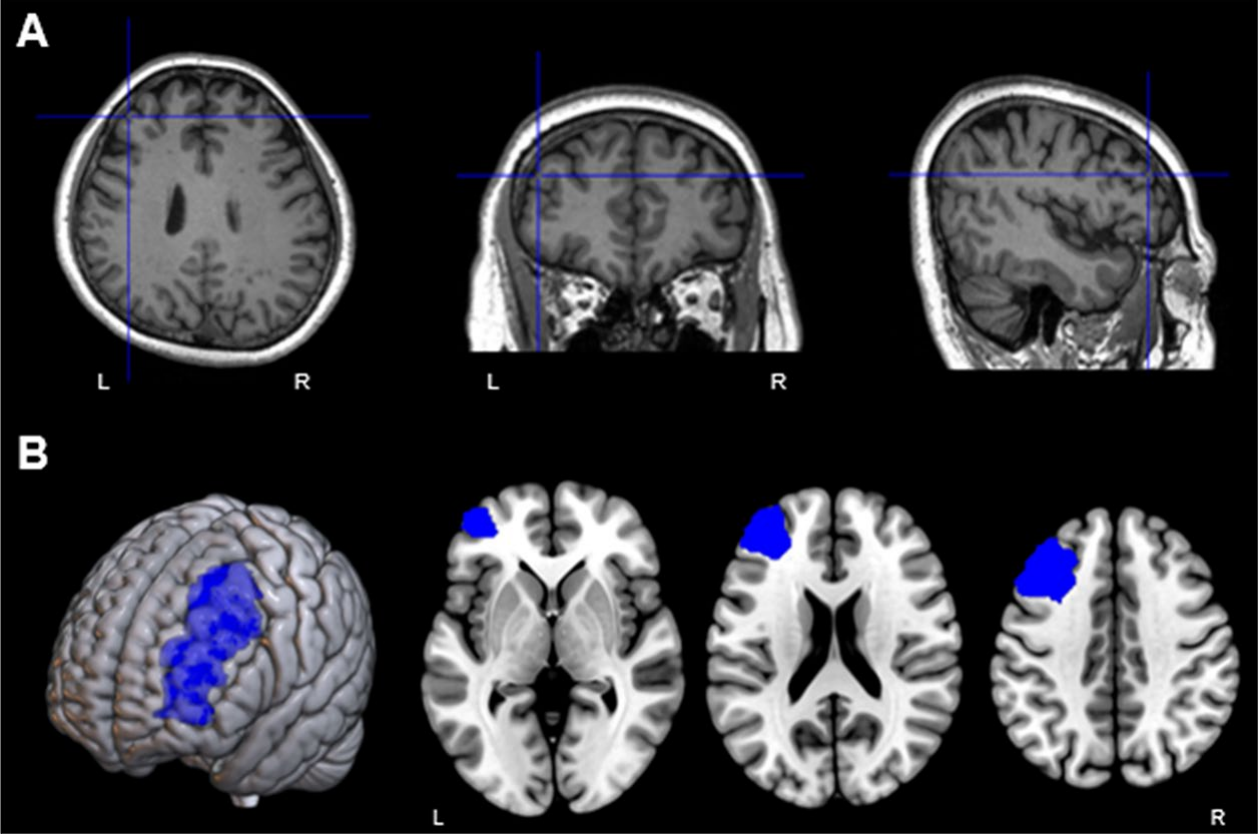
(a) Target brain region for the robotic repetitive transcranial magnetic stimulation (rTMS) treatment of a patient. (b) Region of interest for single-photon emission computed tomography (SPECT) analysis.
